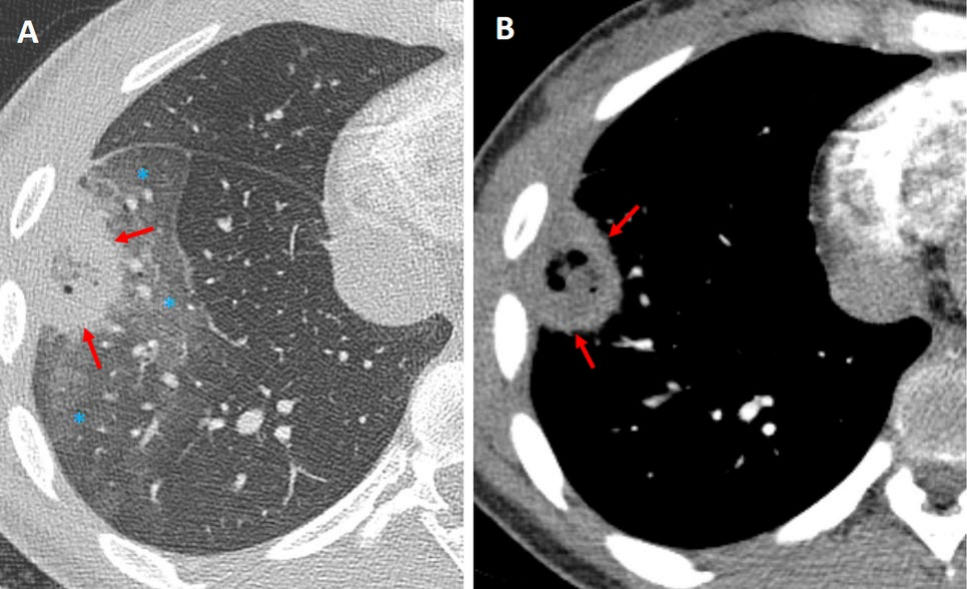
Atoll sign (arrows) in a patient with pulmonary paragonimiasis. Chest CT images with (A) lung window and (B) mediastinal window settings demonstrate a solitary, right lower lobe lateral basilar, subpleural irregular pulmonary opacity with the atoll sign (arrows) and surrounding ground-glass opacity (*). Note the adjacent pleural thickening
